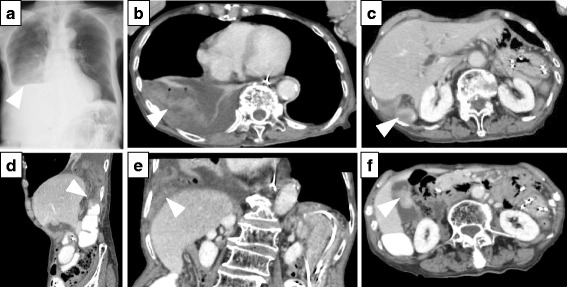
Radiographic images of the adult right-sided Bochdalek hernia prior to surgery. a Chest X-ray showed intestinal gas over the liver and an elevated right hemi-diaphragm (white arrow head). b A computed tomography (CT) scan of the abdomen and pelvis demonstrated reduced contrast uptake and thickening of the herniated small intestinal wall. The herniated small intestine was present in the thorax (white arrow head). c CT scan of the abdomen and pelvis demonstrated herniation of the small intestine into the right thoracic cavity from the posterior surface overlying the right hepatic lobe (white arrow head). It also demonstrated reduced contrast uptake and thickening of the herniated small intestinal wall. The liver was not atrophic. d A sagittal sequence of CT scans confirmed herniation of the small intestine into the right thoracic cavity from the posterior surface overlying the right hepatic lobe (white arrow head). e A coronal sequence of CT scans also demonstrated herniation of the small intestine into the right thoracic cavity from the posterior surface overlying the right hepatic lobe (white arrow head). f CT scan of the abdomen and pelvis also demonstrated a torose lesion in the gallbladder (white arrow head)
